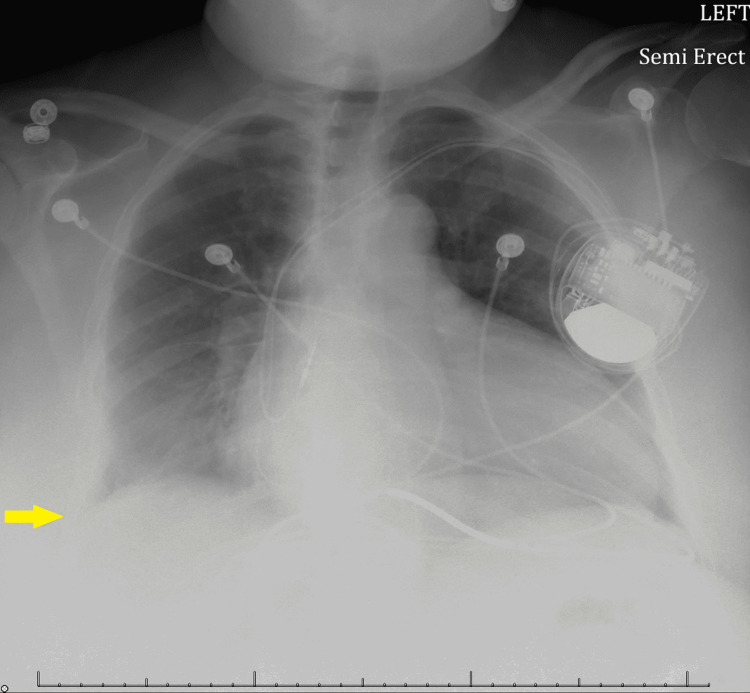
Chest x-ray showing complete resolution of the previous consolidation and pleural effusion on the right side (yellow arrow). Between hospitalizations, an automatic implantable cardioverter defibrillator was inserted for primary prevention of sudden cardiac death in the setting of the patient's severely reduced ejection fraction and New York Heart Association class II functional status.
